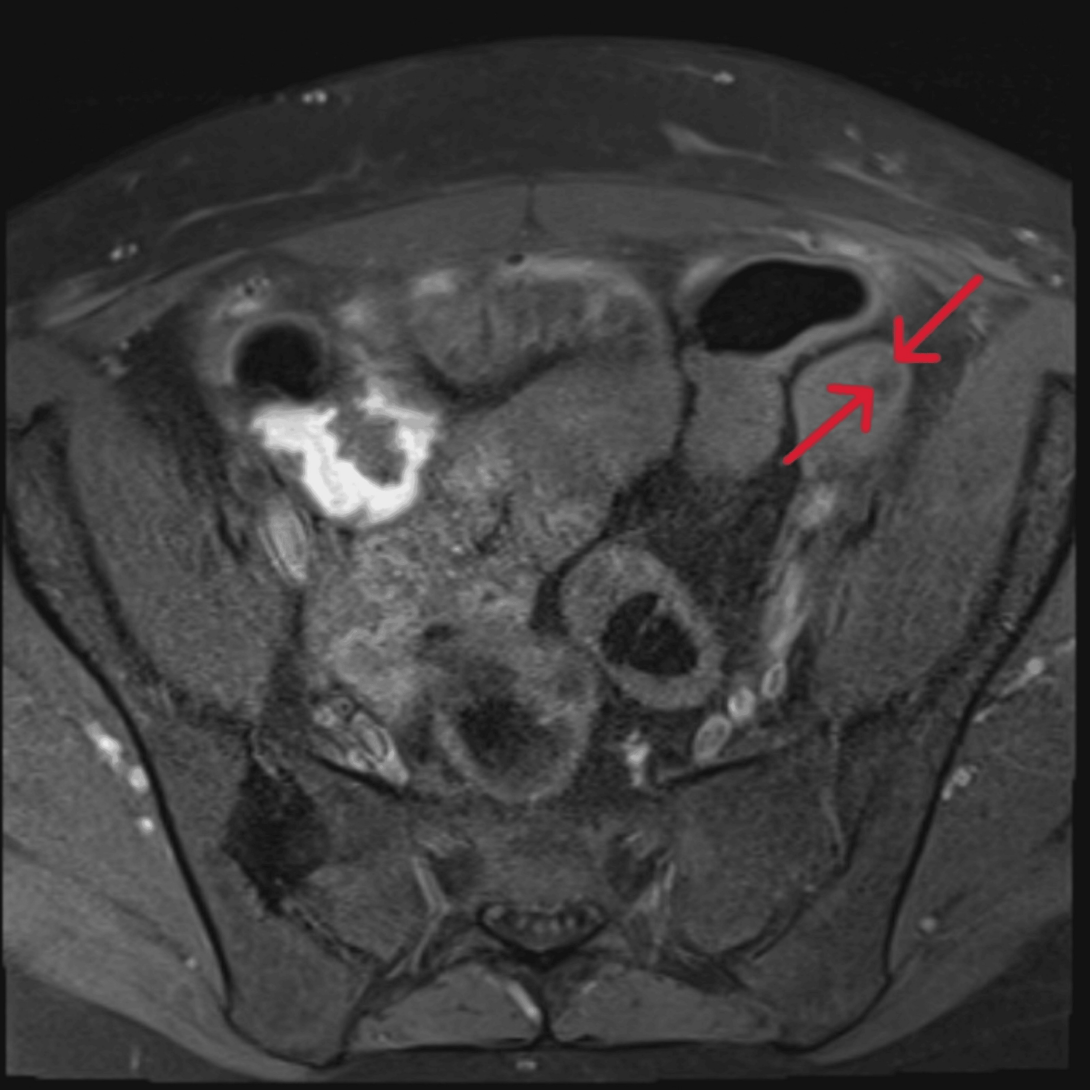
MRI of the pelvis demonstrating left ovarian teratoma Coronal T2-weighted MRI of the pelvis highlights a heterogeneous left adnexal mass (red arrows), consistent with a left ovarian teratoma MRI: magnetic resonance imaging
